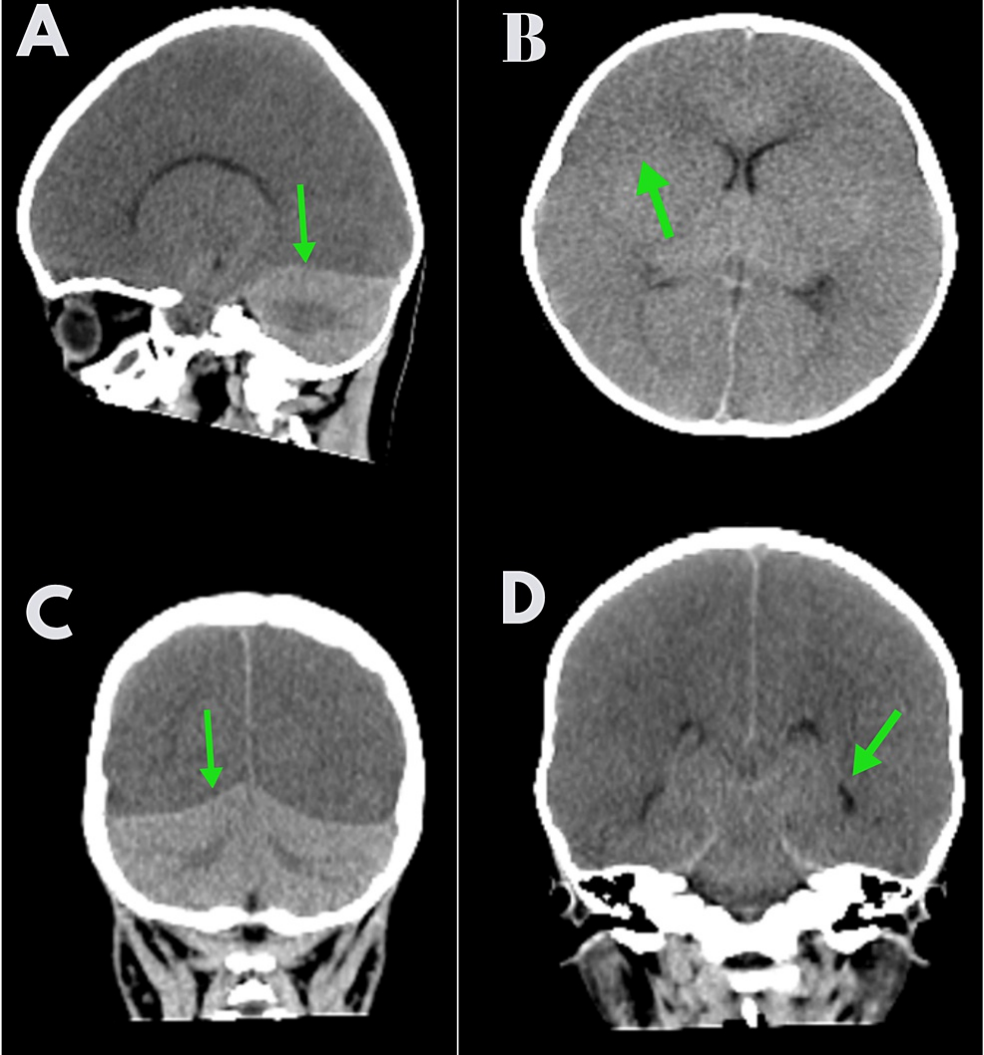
CT head showing diffuse brain edema with hypoxic-ischemic encephalopathy A: Sagittal view showing a generalized decrease in the density of the supratentorial brain due to edema with a relatively normal cerebellum, which appears as a white cerebellum sign; B: Axial view showing diffuse brain edema with loss of gray matter differentiation in cerebral hemispheres; C & D: Coronal reformatted image showing white cerebellum sign and effacement of the basal cisterns.
